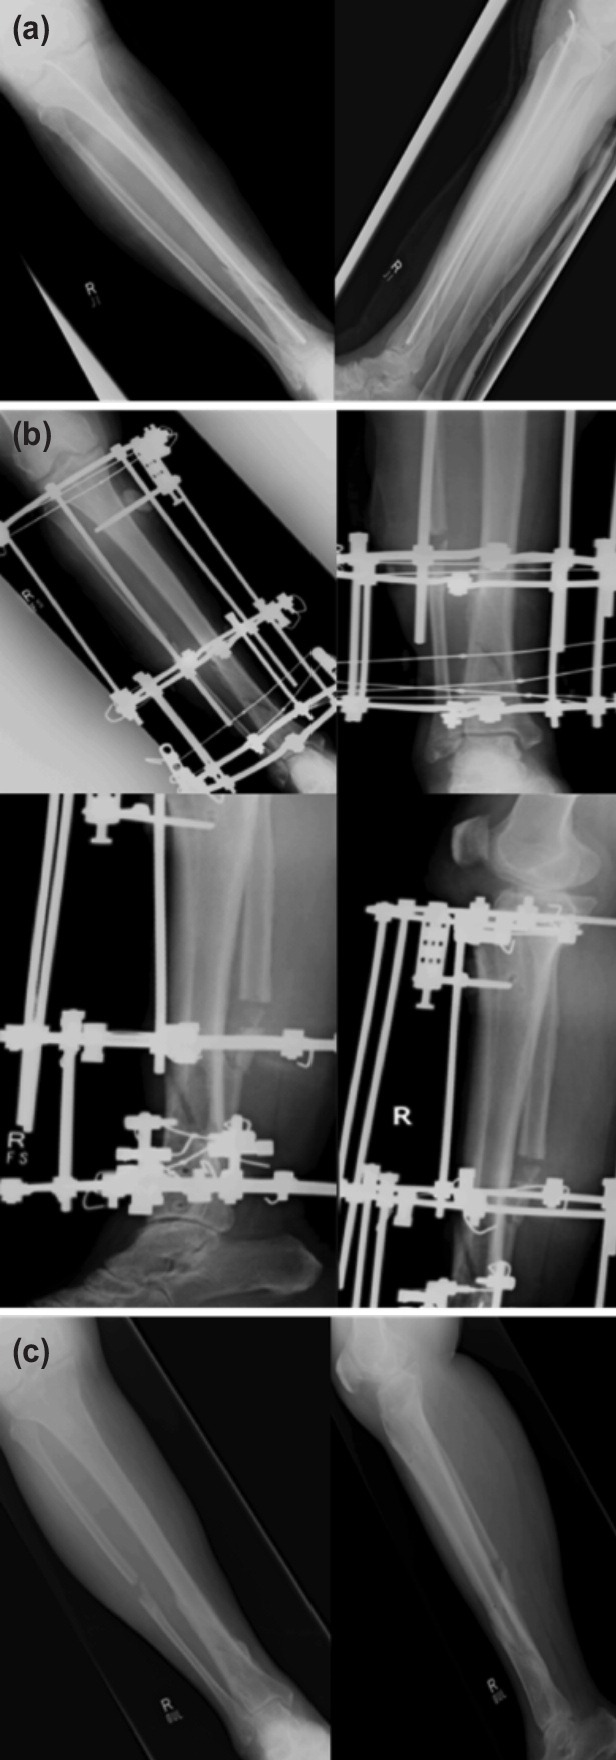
Radiographs of Patient 2. (a) Pre-operative antero-posterior and lateral views showing tibial non-union. (b) Post-operative antero-posterior (top row) and lateral (bottom row) views after Ilizarov application. (c) Antero-posterior and lateral views at final follow-up showing bony union.
